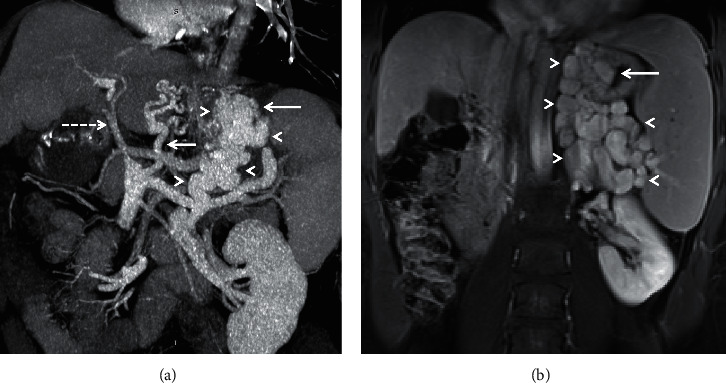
Coronal-oblique MIP (a) and coronal MRI (b) images from two different patients depicting gastrorenal shunts (arrowheads) with varices protruding into the gastric lumen (long solid arrows). *Note.* The attenuated main portal vein (dashed arrow) and prominent left gastric vein (short solid arrow) in (a).
